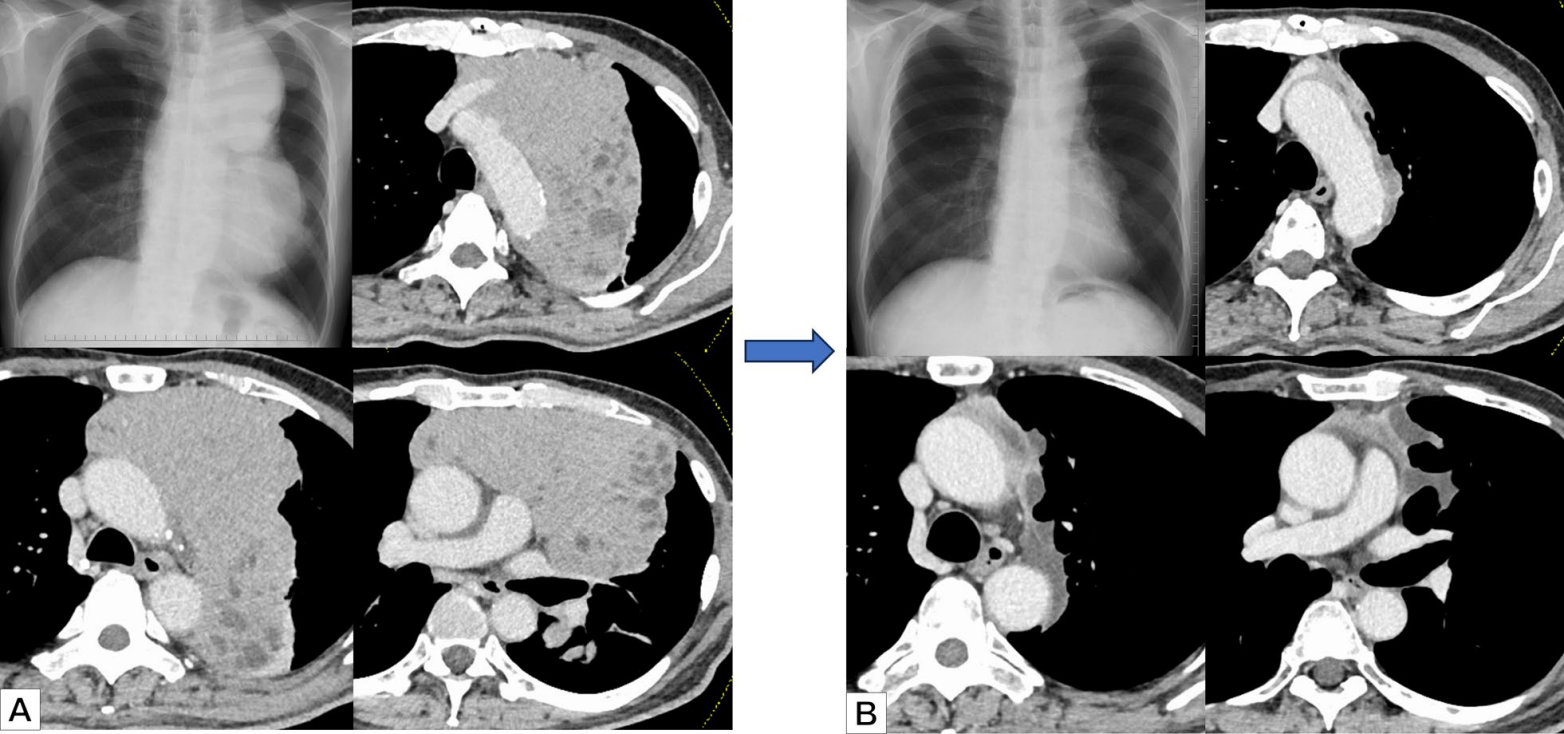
A Before any treatment, chest X-ray and computed tomography images revealed a 21 cm horseshoe-shaped mass in the anterior mediastinum, encircling the pulmonary hilum and extending into the left thoracic cavity. B After four cycles of preoperative induction chemotherapy with cisplatin, doxorubicin, and methylprednisolone (CAMP), chest X-ray and computed tomography images revealed tumor shrinkage to 12 cm and a partial response
